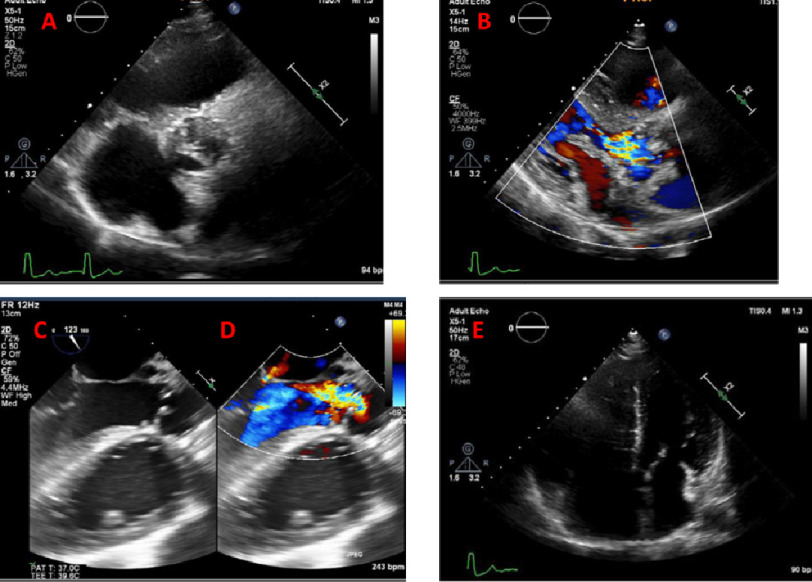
Trans-thoracic echo, short axis view at level of aorta showing calcified leaflets with restricted closure and mal-coaptation (A). Trans-thoracic echo, parasternal long axis view showing color Doppler of the aortic valve with severe aortic regurgitation (B). Transesophageal echo, 120-degree view showing the aortic valve with restricted calcific leaflets in 2D (C) and color Doppler showing severe aortic regurgitation (D). Trans-thoracic echo, apical four chamber view showing dilated hypertrophied RV due to pressure overload (E).
